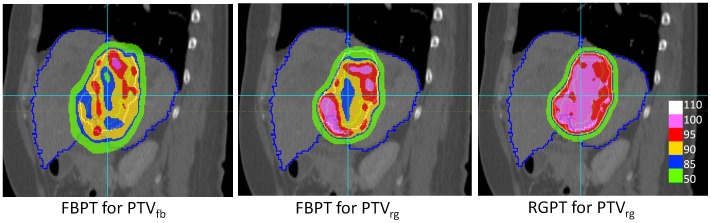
Comparison of dose distribution between FBPT and RGPT. Images of the dose distributions with FBPT for PTV_fb_(left), FBPT for PTV_rg_ (center), and RGPT for PTV_rg_ (right) for the CT images of patient B with tumor motion ID of b.
